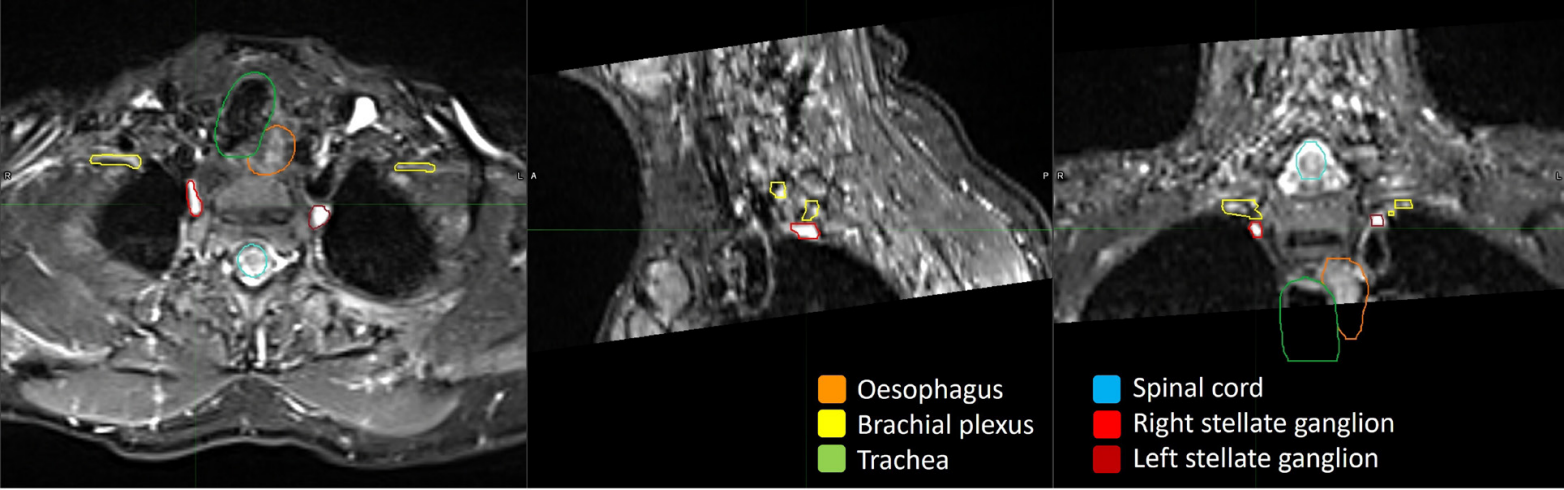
1.5T MRI diagnostic imaging of the stellate ganglia. A T2 weighted turbo spin echo MRI with short tau inverted recovery fat suppression of a participant with a left sided ICD device. Planning target volumes are contoured over the stellate ganglia and key dose-limiting organs. ICD, implantable cardioverter defibrillator.
